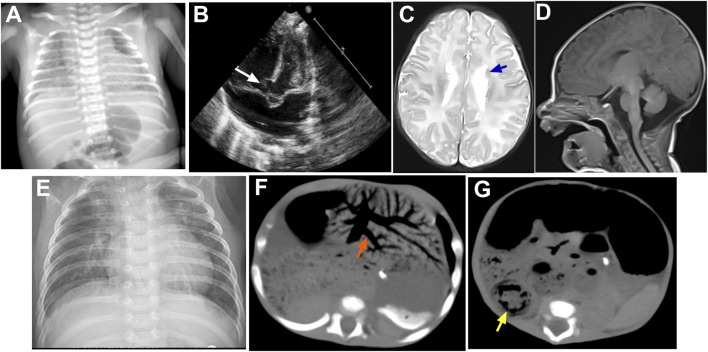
Imaging findings of the patient. (A) Chest X-ray demonstrating bilateral diffuse decreased lung lucency; (B) transthoracic echocardiography demonstrating perimembranous VSD (white arrow); (C,D) cerebral MR imaging demonstrating bilateral PNH (blue arrows) and a large mega cisterna magna; (E) chest X-ray showing interstitial changes; (F,G) abdominal CT demonstrating extensive hepatic portal venous gas (orange arrow) and intestinal wall thickening (yellow arrow). Abbreviations: VSD, ventricular septal defect; PNH, periventricular nodular heterotopia.
